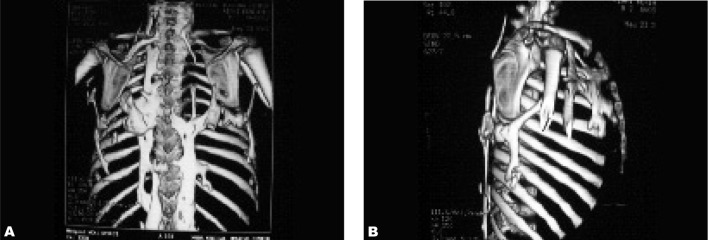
VR and 3D reconstructed CT of thoracic bones shows ossification in the paravertebral and periscapular region and the right arm in the same case at 2 years of age
